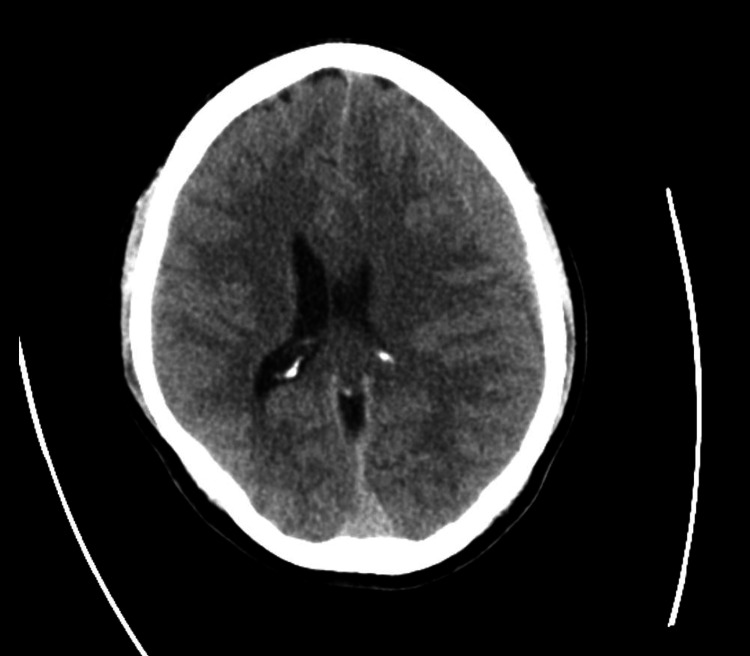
First CT of the brain showing bilateral chronic SDH, measuring 1.5 cm in thickness on the left and 0.5 cm on the right.
